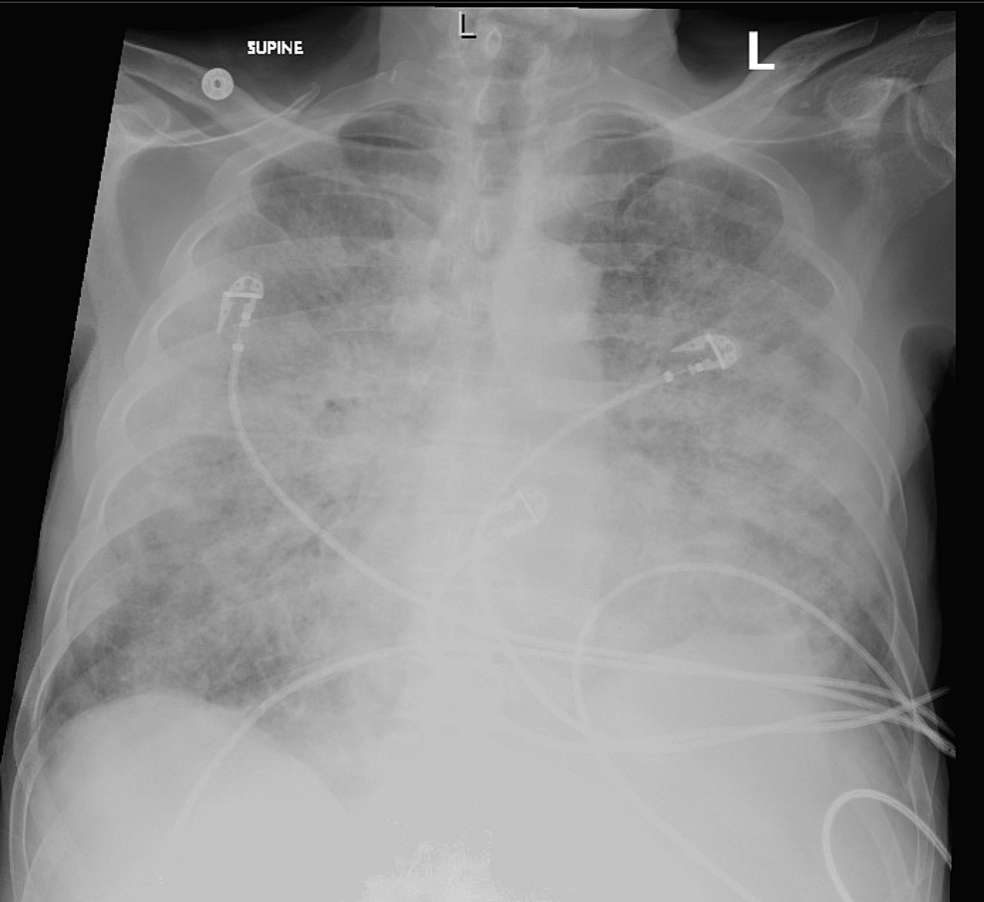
Chest X-ray four days after the beginning of symptoms and discontinuation of azacitidine
